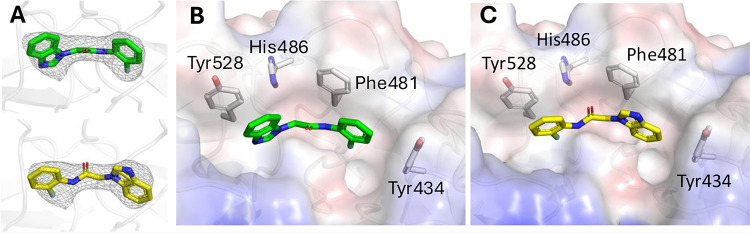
X-ray structure of 1 bound to KLHL12 (9Y8J).
